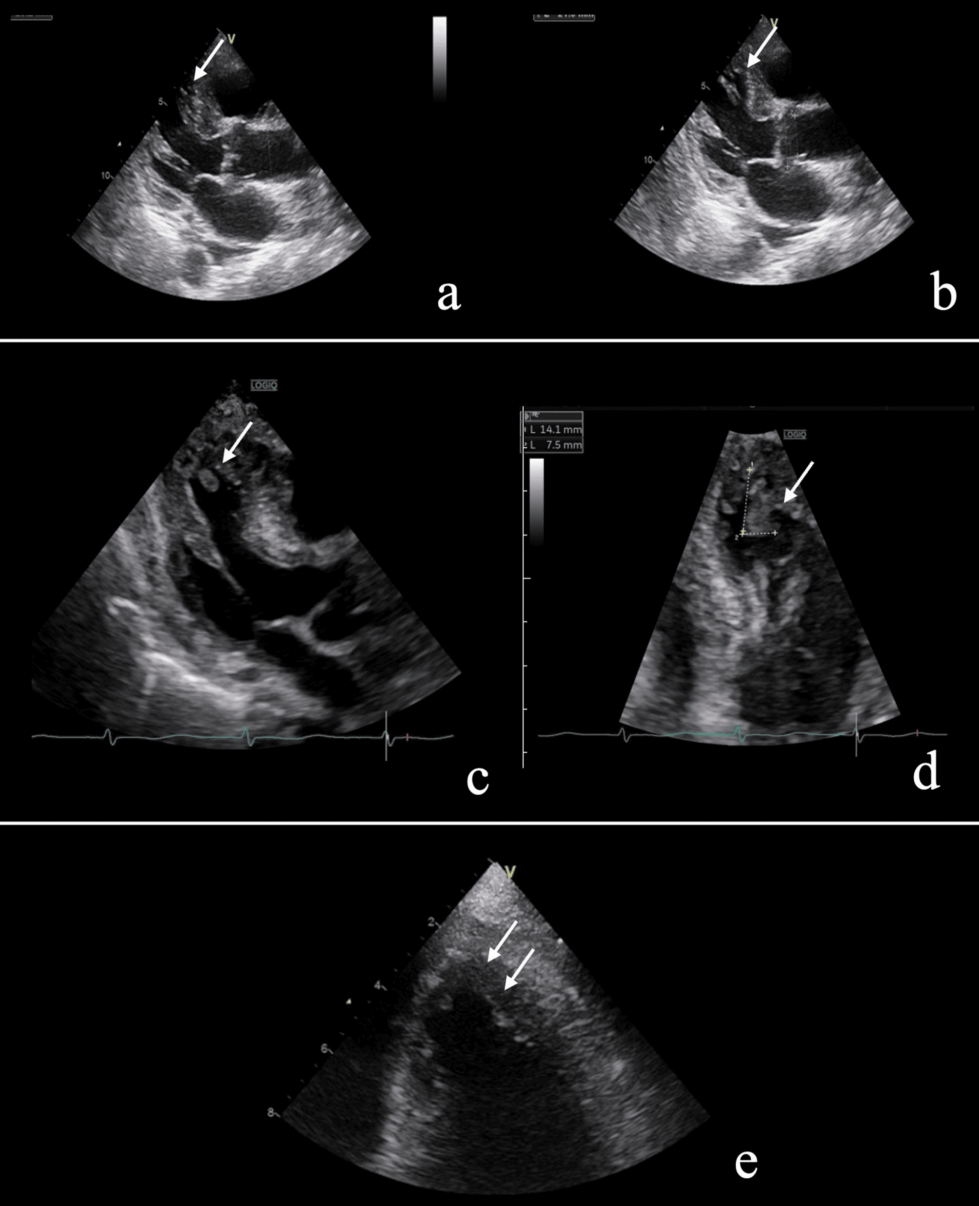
Transthoracic echocardiography (TTE) findings (a) Long-axis view during systole on initial examination. The apical region shows no systolic contraction (arrow), and no definite vegetations are identified. (b) Long-axis view on initial examination during diastole. The apex is the same as during systole (arrow). (c) Follow-up long-axis view on hospital day 13 demonstrating a newly developed apical vegetation on long-axis view (arrow). (d) Details of apical vegetation on hospital day 13 (arrow). It measures 14 × 7 mm, is highly mobile, and has an irregular surface. (e) On TTE performed immediately after the cerebral infarction was identified, the vegetation had completely disappeared (arrow)
